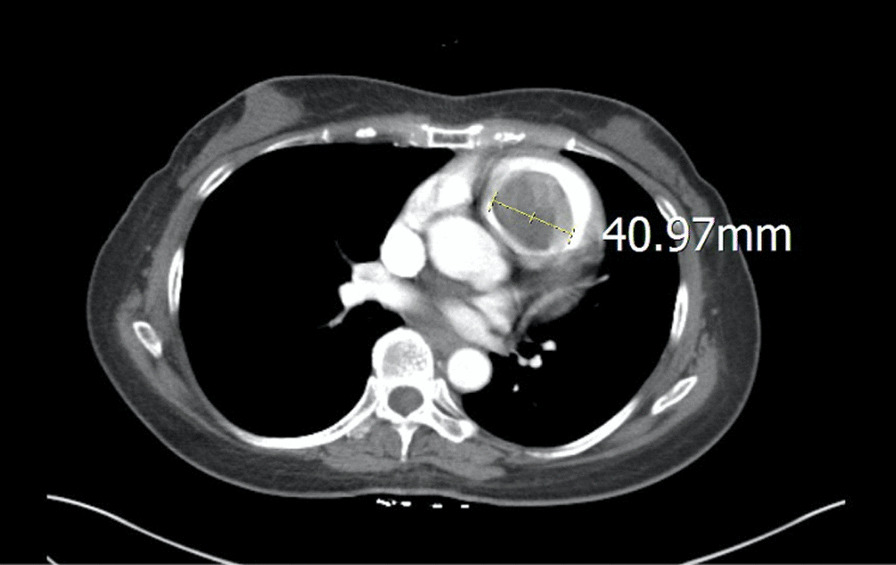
CT scan showing a huge myxoma originating from the tricuspid valve and extending into the right ventricular outflow tract and pulmonary trunk, with a maximum diameter of about 41 mm
